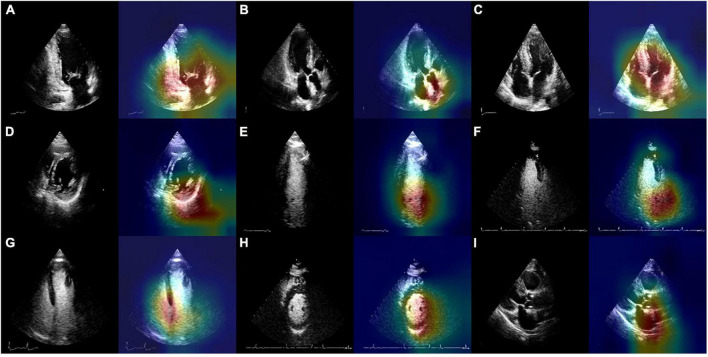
Original images and the results of EchoV-Net visualization of the most related regions for view recognition. (A) 2DE.A2C, (B) 2DE.A3C, (C) 2DE.A4C, (D) 2DE.PSAX, (E) C2DE.A2C, (F) C2DE.A3C, (G) C2DE.A4C, (H) C2DE.PSAX, and (I) Other. 2DE, two-dimensional echocardiography; C2DE, contrast two-dimensional echocardiography; A2C, apical 2-chamber; A3C, apical 3-chamber; A4C, apical 4-chamber; PSAX, parasternal left ventricular short axis; Other, including parasternal left ventricular long axis, pulmonary artery long axis, and major artery short axis.
